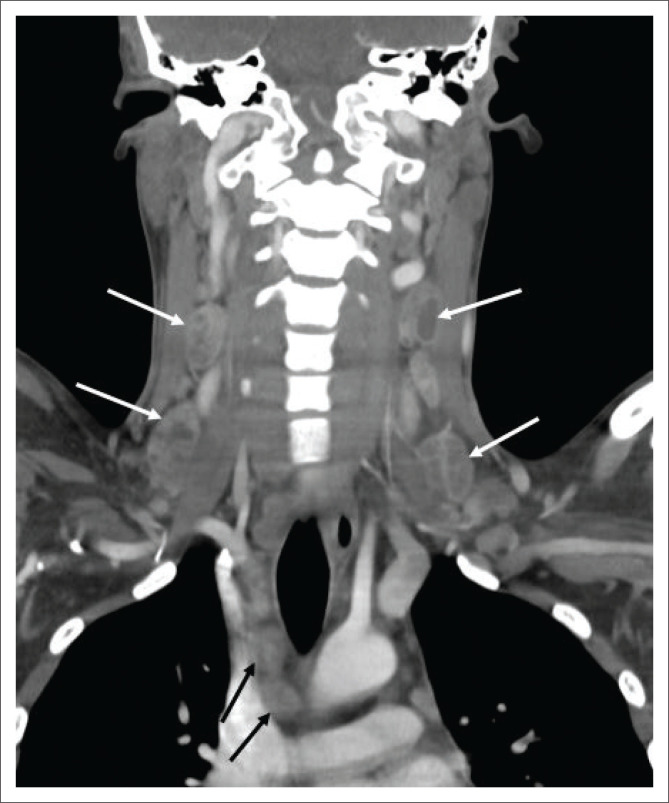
A 24-year-old human immunodeficiency virus-negative male with aplastic anaemia presented with bilateral neck swelling. Lymph node biopsy confirmed tuberculous lymphadenitis. Coronal post-contrasted computed tomography of the neck demonstrated multiple, bilateral cervical (solid white arrows) and paratracheal (solid black arrows) lymph nodes with necrotic centres consistent with tuberculous lymphadenitis.
